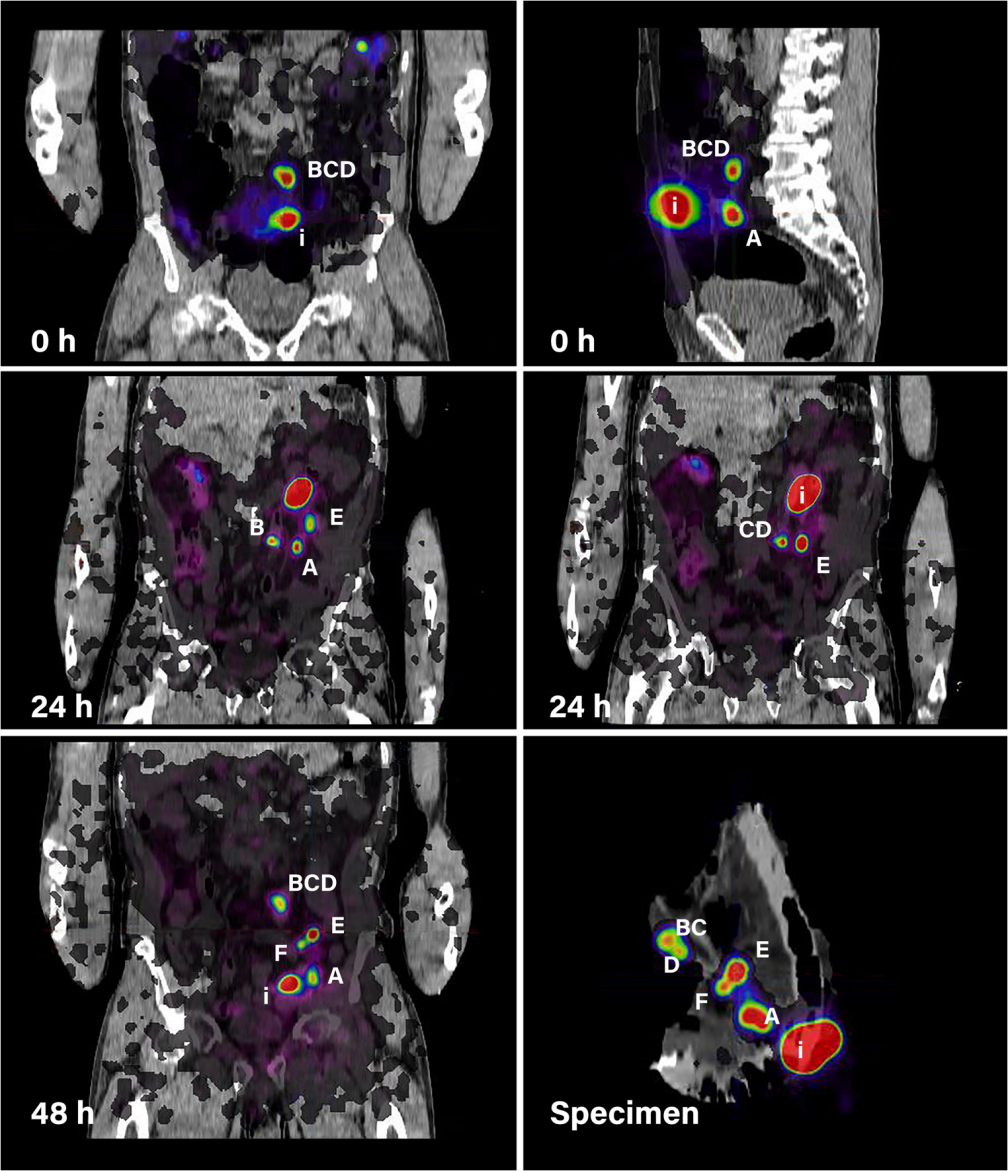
SLN identification at PET/CT images of patient 2. Injection side is shown as `i` in all images. Foci A, B, C and D showed in total five sentinel lymph nodes and were already apparent at first scan (0 h after injection). Foci E represented two sentinel lymph nodes first seen at the second PET/CT scan (24 h after injection) and F was first seen at the third scan after 48 h. Lymph nodes E and F were probably hidden behind the other 'hotter' nodes. All SLNs were intraoperatively identified using near-infrared imaging
